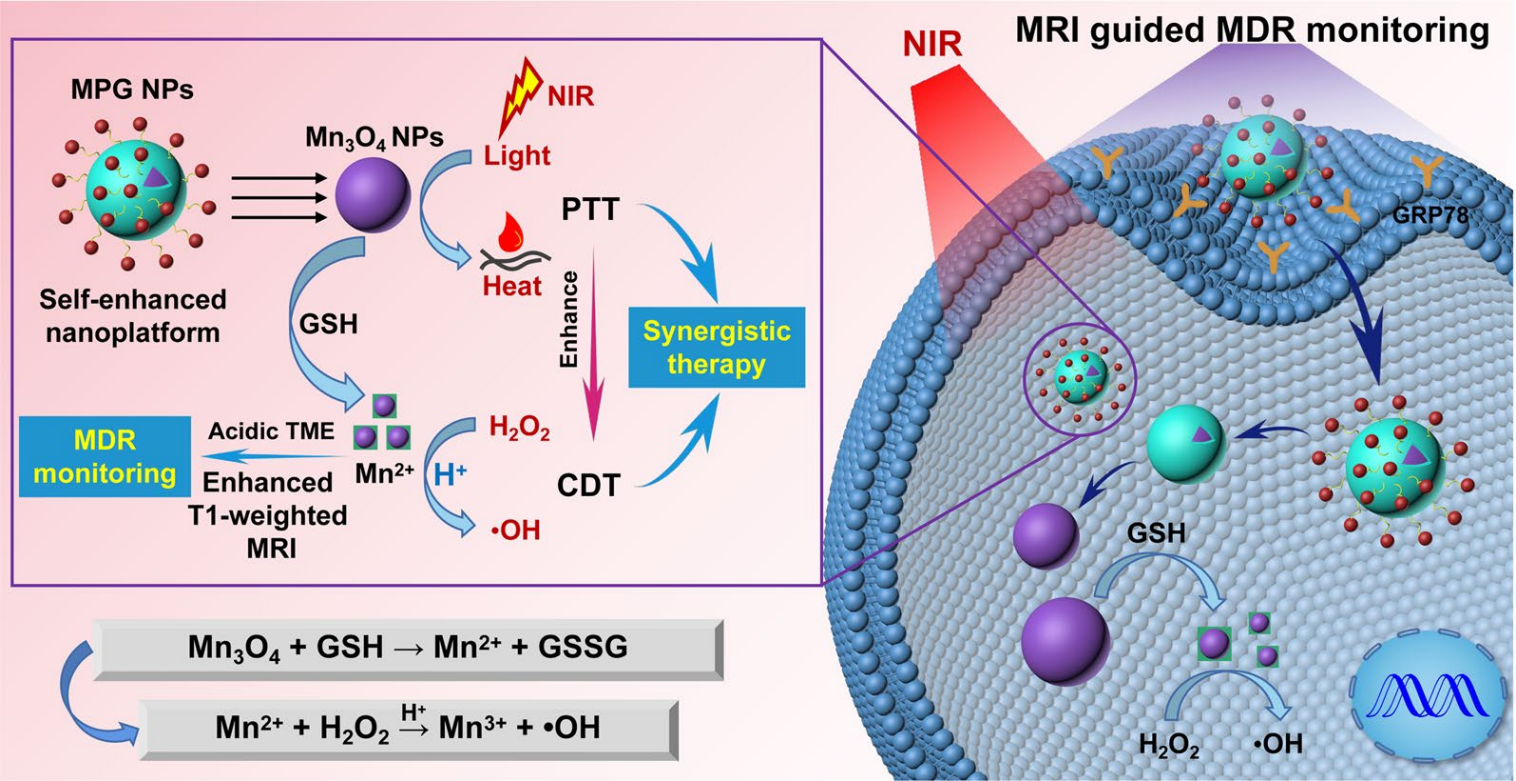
The mechanism of pH/H_2_O_2_/GSH-responsive MPG NPs as a multifunctional self-enhanced nanoplatform for gastric cancer MDR monitoring and CDT/PTT synergistic therapy. After endocytosis, MPG NPs can react with intracellular GSH by redox reaction to produce Mn^2+^, which has Fenton-like activity and can convert endogenous H_2_O_2_ into highly toxic ·OH under HCO_3_^−^ conditions. Mn^2+^ can enhance MRI for in vivo MDR monitoring. Under laser irradiation, MPG NPs can perform CDT/PTT synergistic therapy of MDR in gastric cancer
